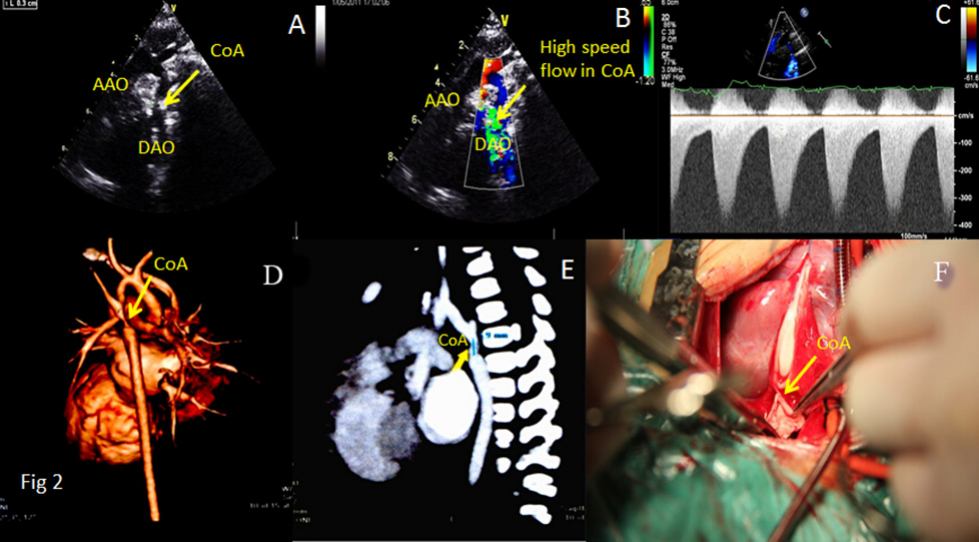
Transthoracic echocardiograms in suprasternal view of a 3-month-old girl showing [A] the location of the CoA, [B] the high flow signal in the CoA, and [C] characteristics of the CoA by continuous wave Doppler, including high speed jet spectrum during systole and extended speed reduction during diastole. Computed tomographic angiography [D,E] showed the location of the CoA. At surgery [F], the location of the CoA was confirmed. Abbreviations as in Fig 2.
